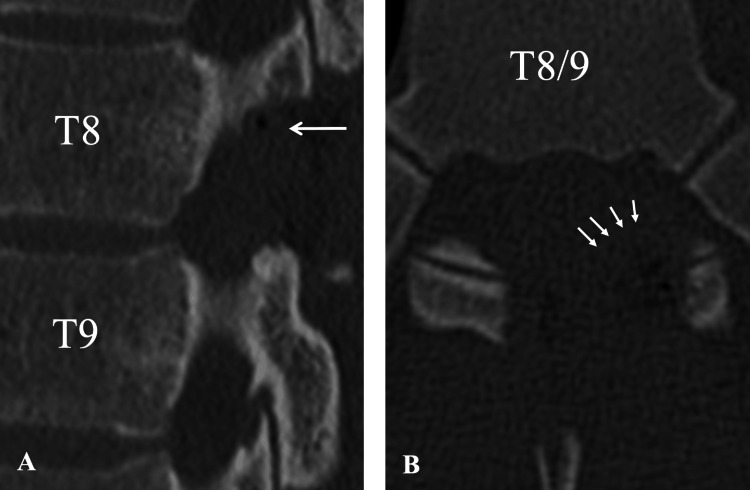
Postoperative CT images following left-sided partial laminectomy and resection of the ossified ligamentum flavum at T8-T9 A) Sagittal CT view demonstrating restoration of the intervertebral foramen with no residual ossification (arrow); B) Axial CT image confirming complete resection of the ossified ligamentum flavum and decompression of the foraminal stenosis at T8-T9 (arrows) CT - computed tomography
